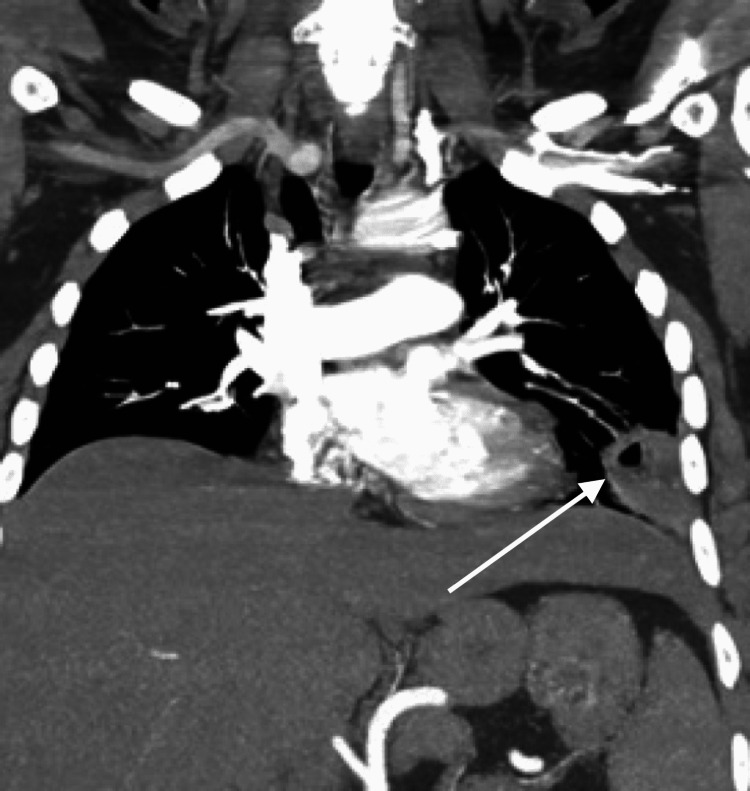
Chest CT (coronal view) demonstrating left lower lobe cavitary lesion.
